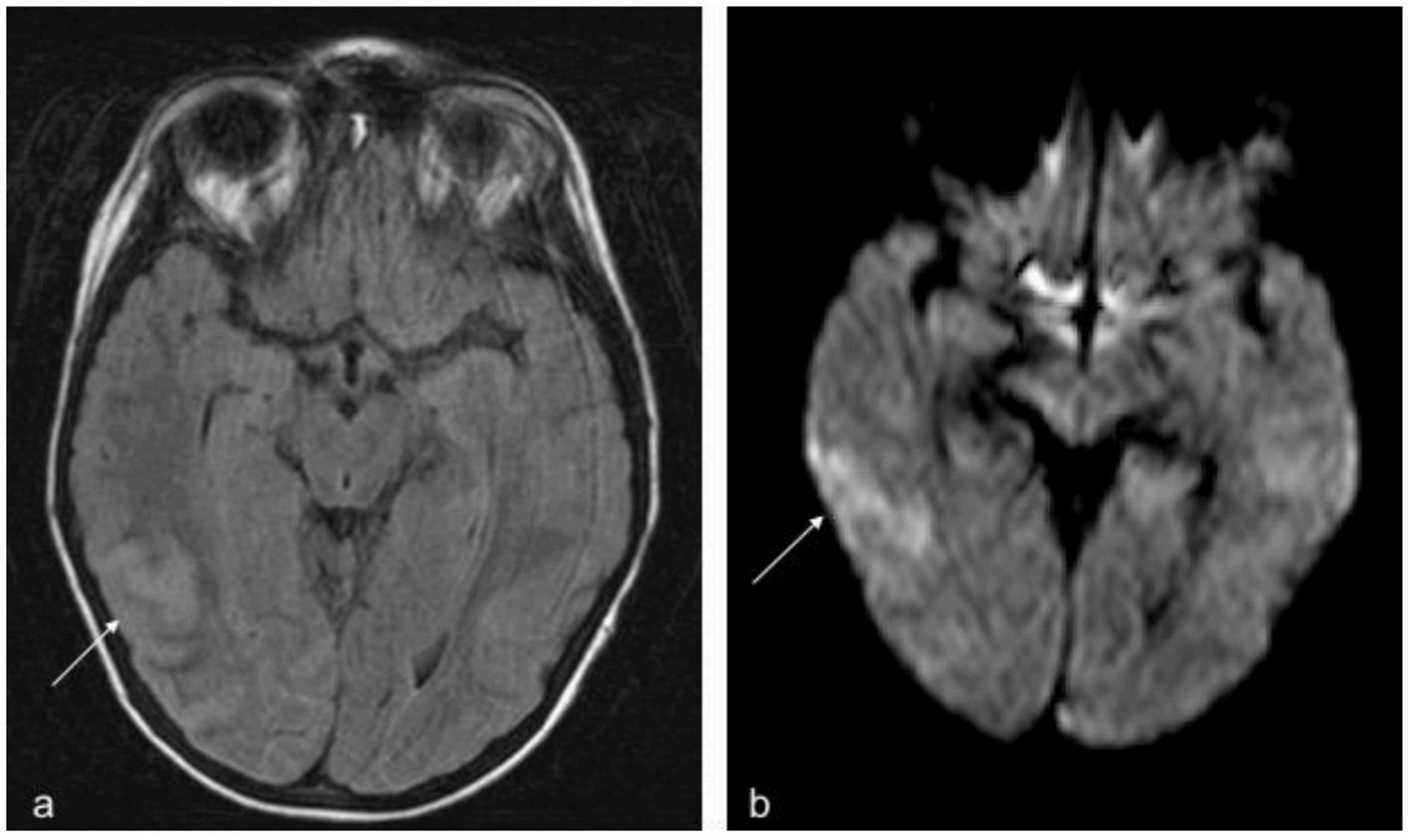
(a) Transverse plane, FLAIR sequence, movement artifacts. The arrow shows the cortical-subcortical lesion compatible with a non-haemorrhagic infarction in a venous territory. (b) Transverse plane, b1000 diffusion sequence. The arrow shows parenchymal shine due to diffusion restriction indicative of acute vascular disease (Not shown ADC map with signal drop in the area).
